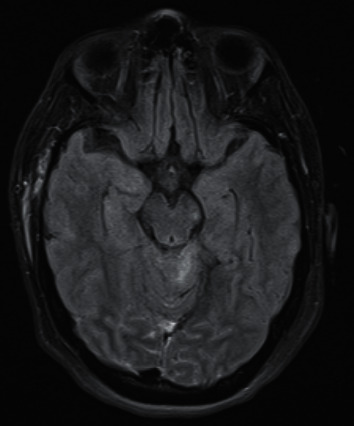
Axial FLAIR MRI, showing Grade III DAI with hyperintense lesions evident in the left midbrain and pons.
